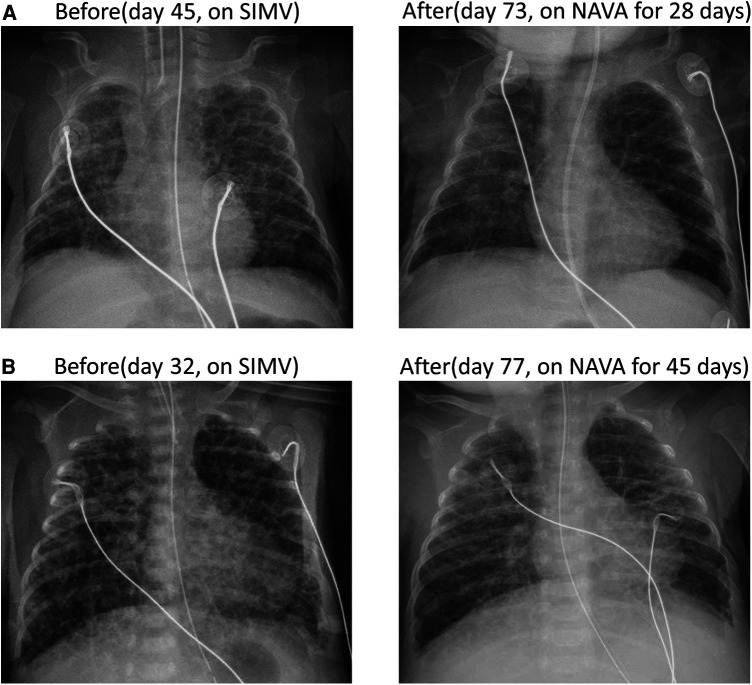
Two cases of diffuse PIE were observed through anterior-posterior chest x-ray before and after NAVA. (A) In case 3, diffuse PIE was observed on day 45 and resolved on day 73 after 28 days of NAVA and NIV NAVA treatment. (B) In case 4, diffuse PIE was detected on day 32 and resolved on day 77 after 45 days of NAVA and NIV NAVA treatment.
